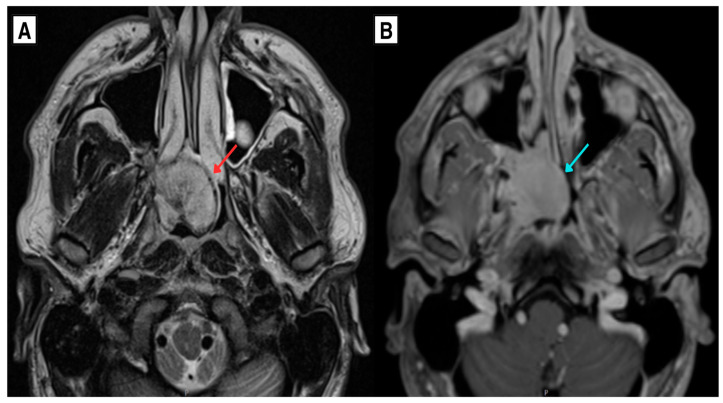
MRI of the nasopharyngeal region. (A) Axial T2-weighted image demonstrates a heterogeneous mass centered in the right nasopharynx (red arrow) with internal hypointense foci and extension into the right pterygopalatine fossa (blue arrow), associated with bony remodeling. (B) Axial post-contrast T1-weighted image demonstrates avid enhancement, consistent with a hypervascular lesion.
